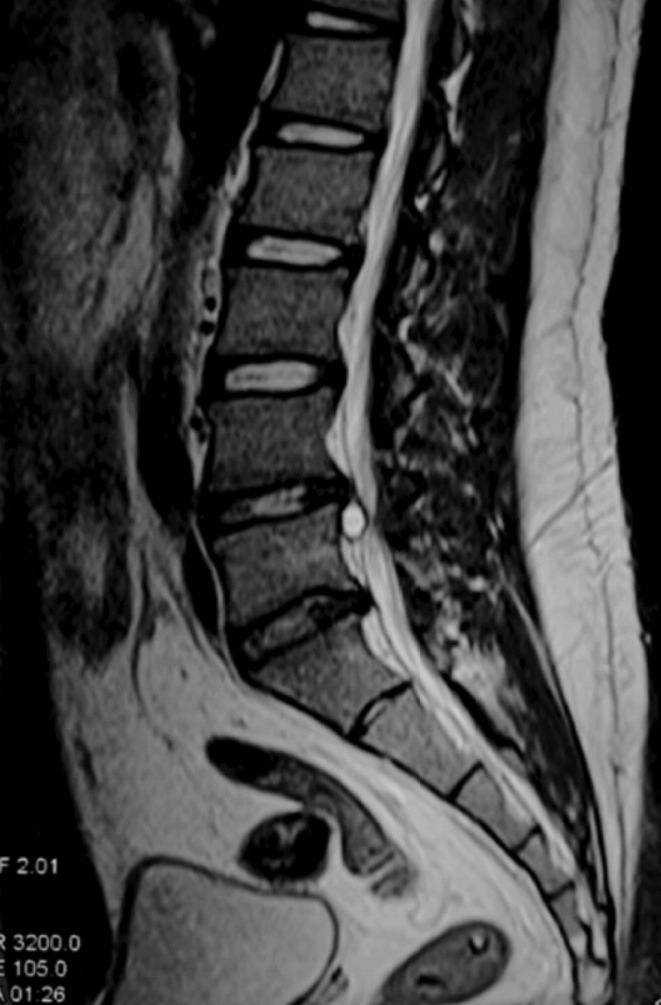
Sagittal view of the lumbar spine MRI showing a cystic lesion in the anterior epidural space with high signal intensity on T2-weighted image.
